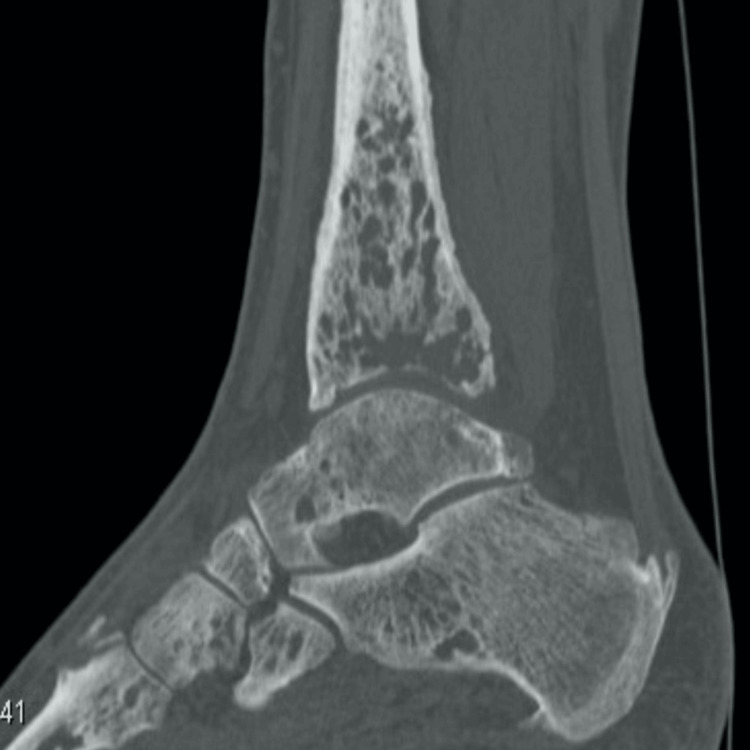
Lateral view of the right foot and tibia on CT.
